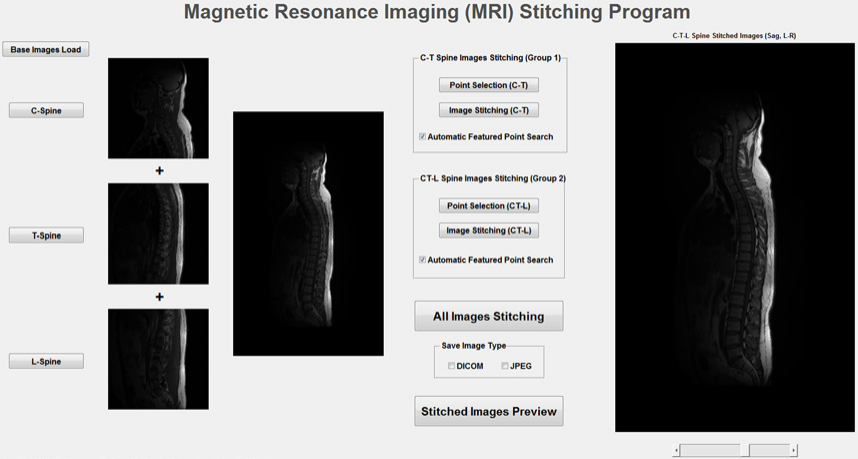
Developed GUI configuration program for C-T-L spine MRI stitching.
